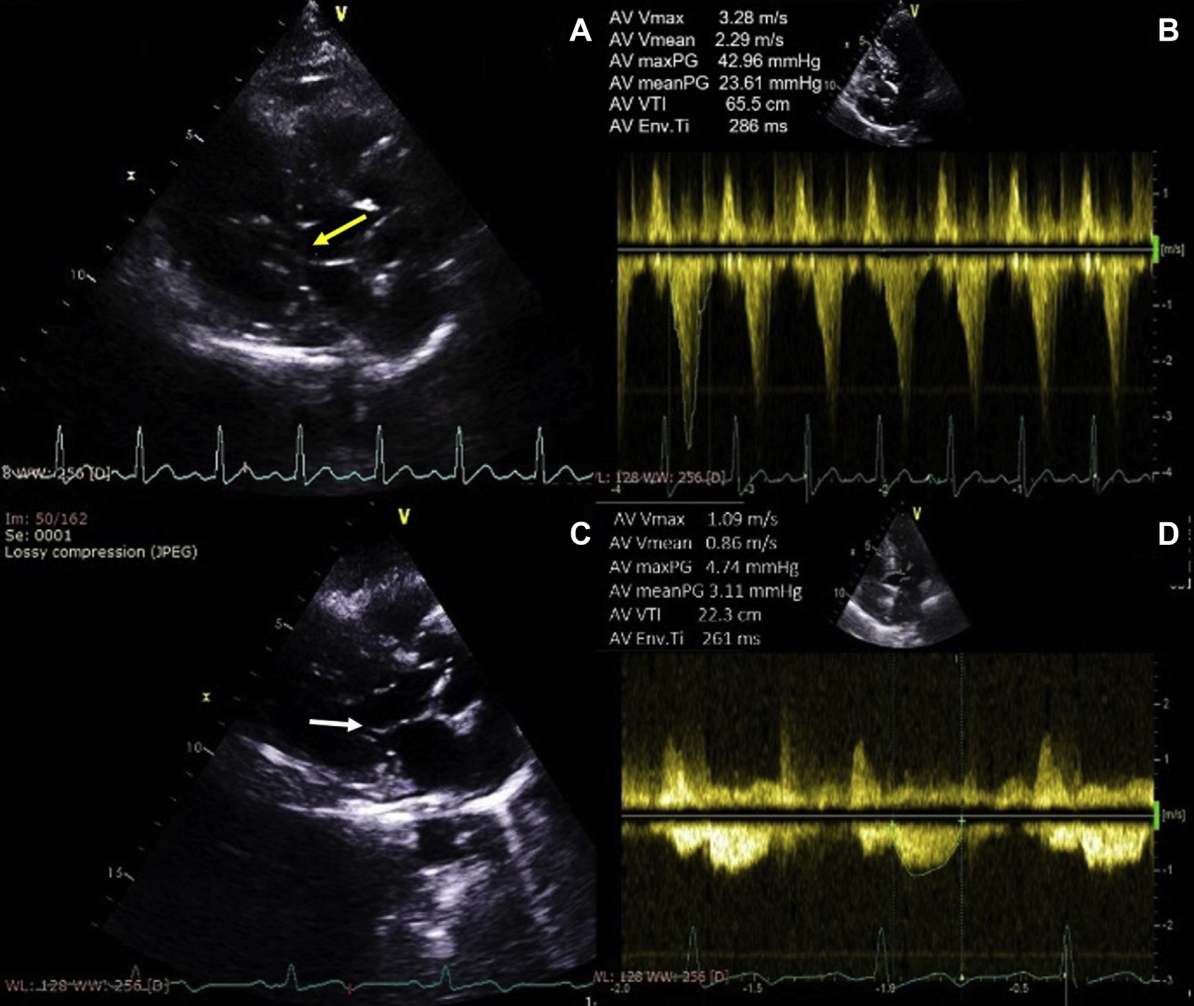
(A) Two-dimensional TTE parasternal long-axis view in systole showing systolic anterior motion of the mitral leaflet (*yellow arrow*) approximating with the basal septum. (B) Two-dimensional TTE apical 5-chamber view in systole with continuous-wave Doppler across the LVOT showing a late systolic jet with a peak gradient of 43 mm Hg. (C) Two-dimensional TTE parasternal long-axis view in systole showing resolution of systolic anterior motion of the mitral leaflet (*white arrow*) after initiating beta-blockers and revascularization. (D) Two-dimensional TTE apical 5-chamber view with continuous-wave Doppler across the LVOT showing a reduction of the peak gradient to 5 mm Hg after initiating beta-blockers and revascularization.
